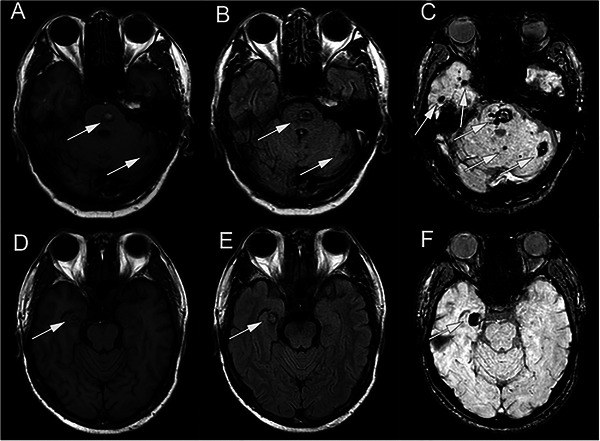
Non‐enhanced brain MRI of familial CCM and sporadic CCM. (A) T1, (B) FLAIR, and (C) SWI sequences of a familial CCM patient with multiple lesions in the pons, cerebellum, and temporal lobe (arrows). (D) T1, (E) FLAIR, and (F) SWI sequences of a sporadic CCM patient with a single lesion in the temporal lobe (arrows).
